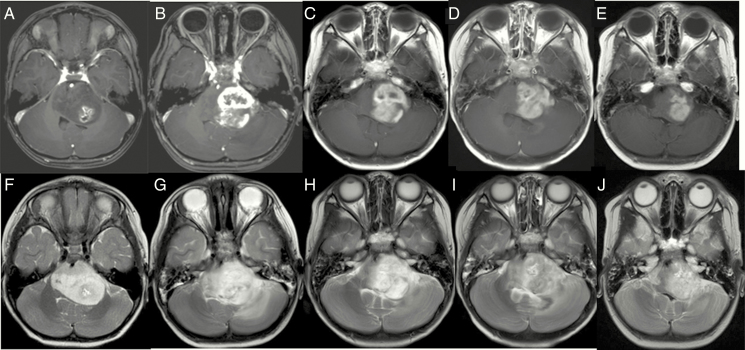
A case of a 3-year-old female treated for DIPG for 6 months prior to starting CED of ACNU (A and F). She was treated with local 54 Gy irradiation plus concomitant TMZ and followed up at another hospital. Due to growing contrast enhancement, she was referred to us and underwent CED of ACNU (B and G). After treatment, TMZ monotherapy was continued. Her symptoms of truncal ataxia, diplopia, and right hemiparesis gradually improved. Images reveal the responses until 3 months after CED of ACNU (C and H: 1 month after CED; D and I: 2 months after CED; E and J: 3 months after CED). (A–E) T1-weighted MRI with contrast enhancement and (F–J) T2-weighted images.
